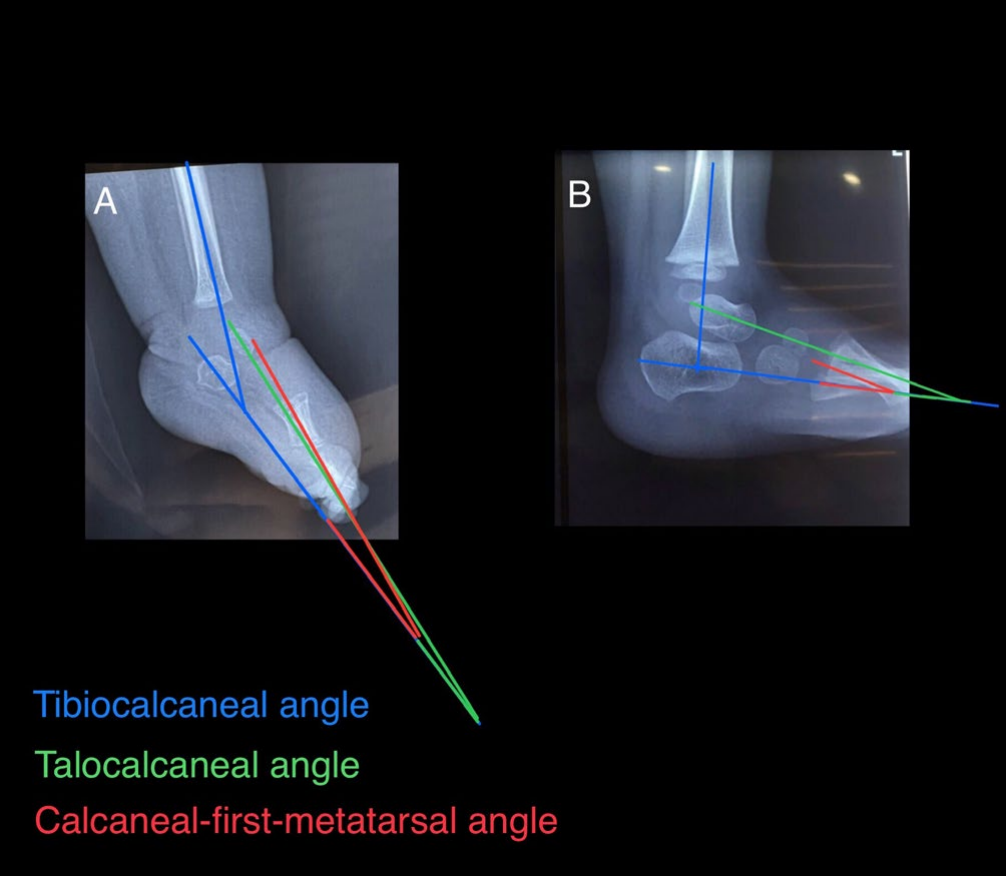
Angle measurements depicted on pre-tenotpmy (A), and one-year post-tenotomy (B) radiographs
